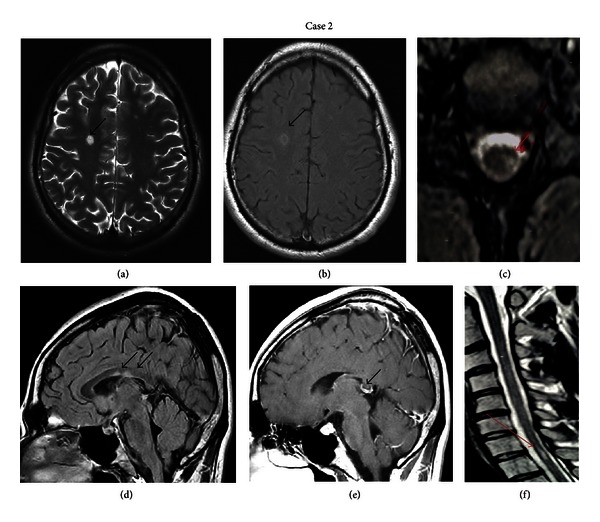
(a) Axial T2- and (b) T1-weighted brain MRI of the second patient showing subcortical hyperintense lesions, one with contrast enhancement (black arrows). (d) and (e) Sagittal T1 weighted with gadolinium brain MRI images showing enhanced corpus callosum lesions (black arrows). (c) Sagittal and (f) axial T2-weighted cervical spine MRI showing one hyperintense lesion (red arrows) on level C7-T1.
